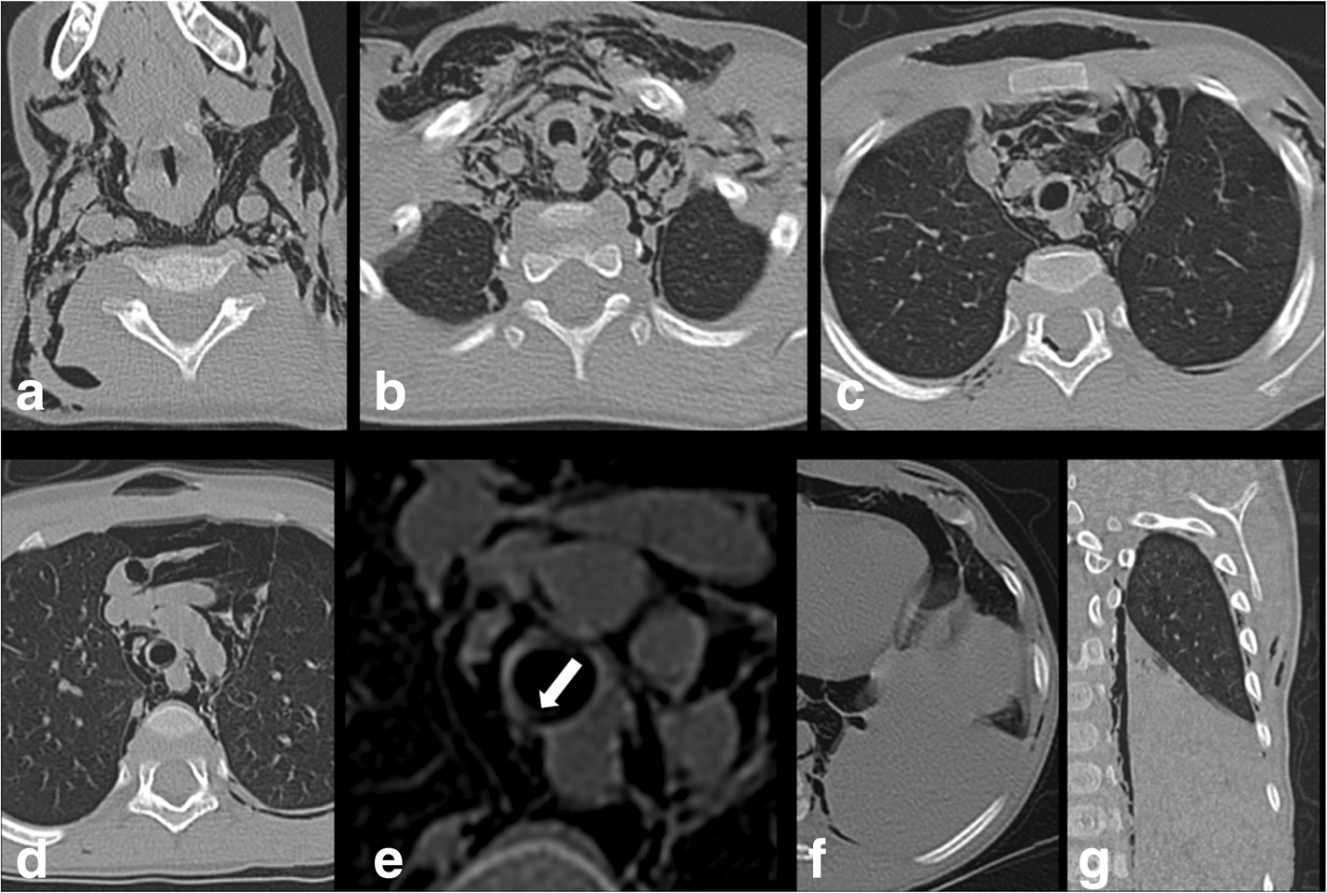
Computed tomography scan of the thorax showed subcutaneous soft tissue emphysema and air dissecting through the neck and mediastinal spaces (a,b). Minimal pneumothorax (b–d), pneumorrhachis within the extradural space in the upper thoracic spine (c), and pneumopericardium (d) were also noted. Thin laceration of the right posterolateral wall of the trachea was detectable (e, *arrow*). Axial and coronal views showed reduction of volume and absence of air bronchogram of the lower lobe of the left lung (f,g)
